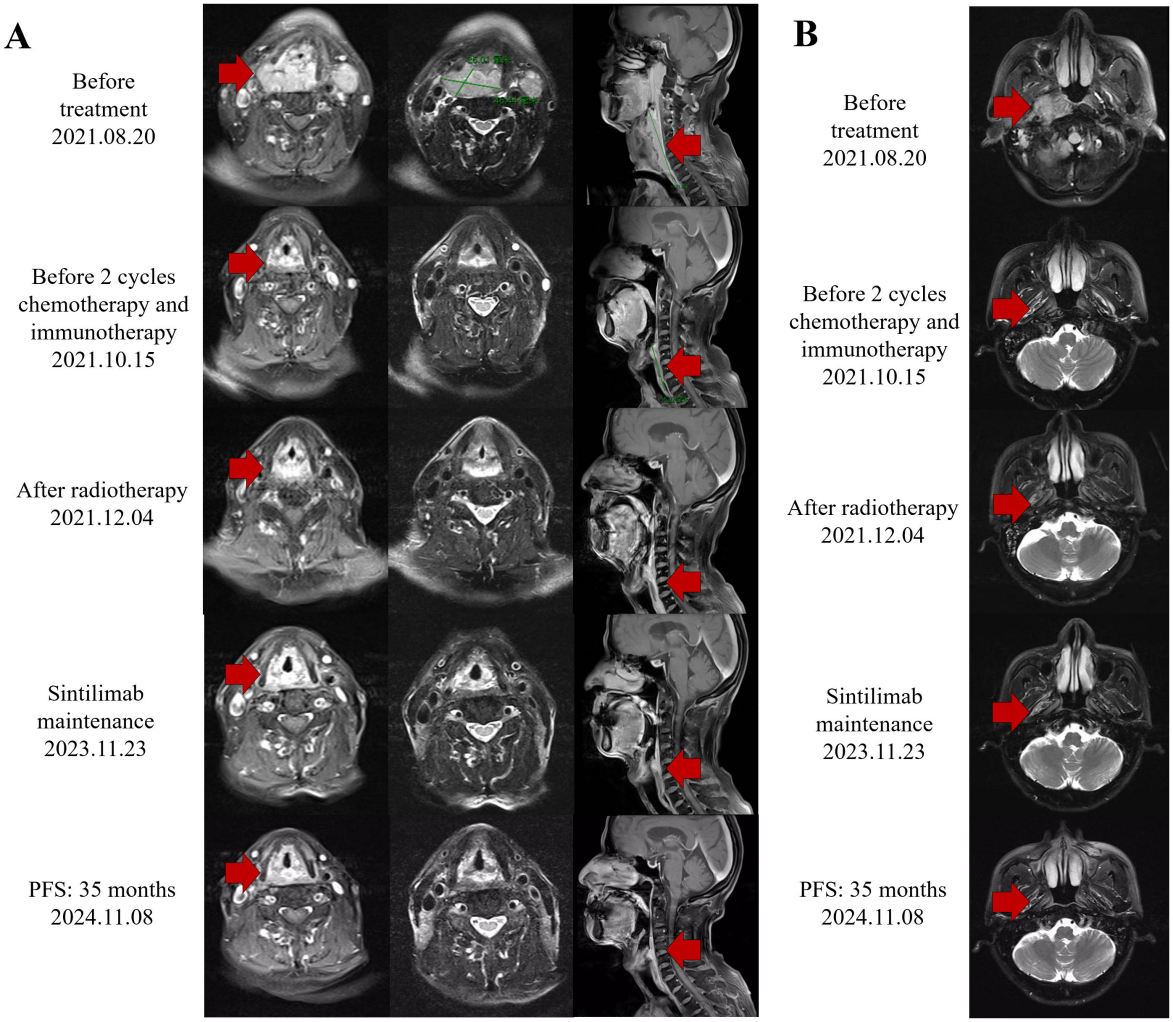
Response evaluation during the clinical course. (A) Representative images of the MRI scan revealed the decreasing process of both hypopharyngeal primary cancer and metastasis lymph nodes. Red arrows indicate tumor lesions. (B) Representative images of the MRI scan revealed the decreasing process of both nasopharyngeal primary cancer. Red arrows indicate tumor lesions.
